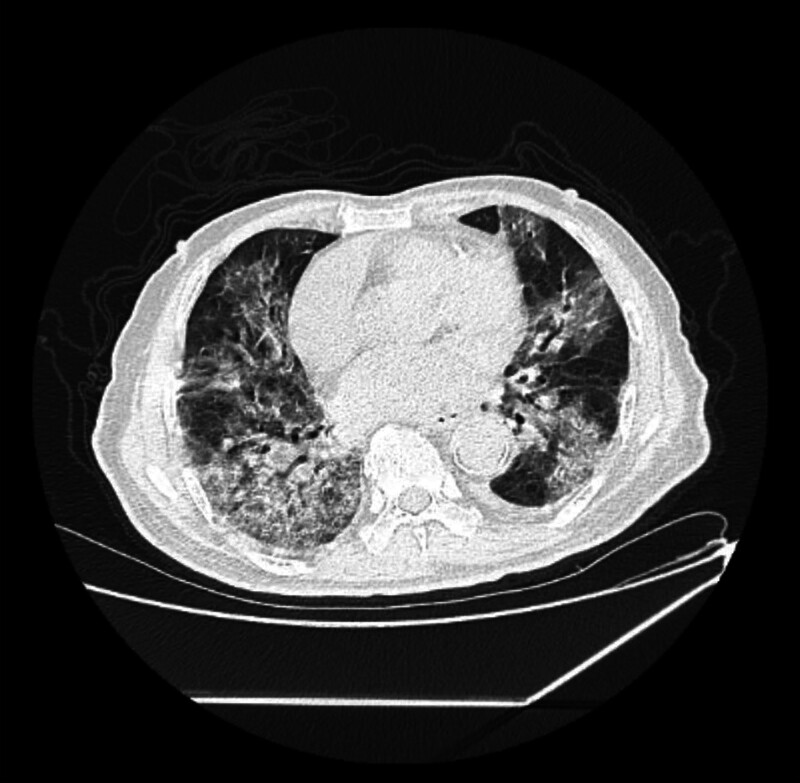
Chest CT image before treatment.
